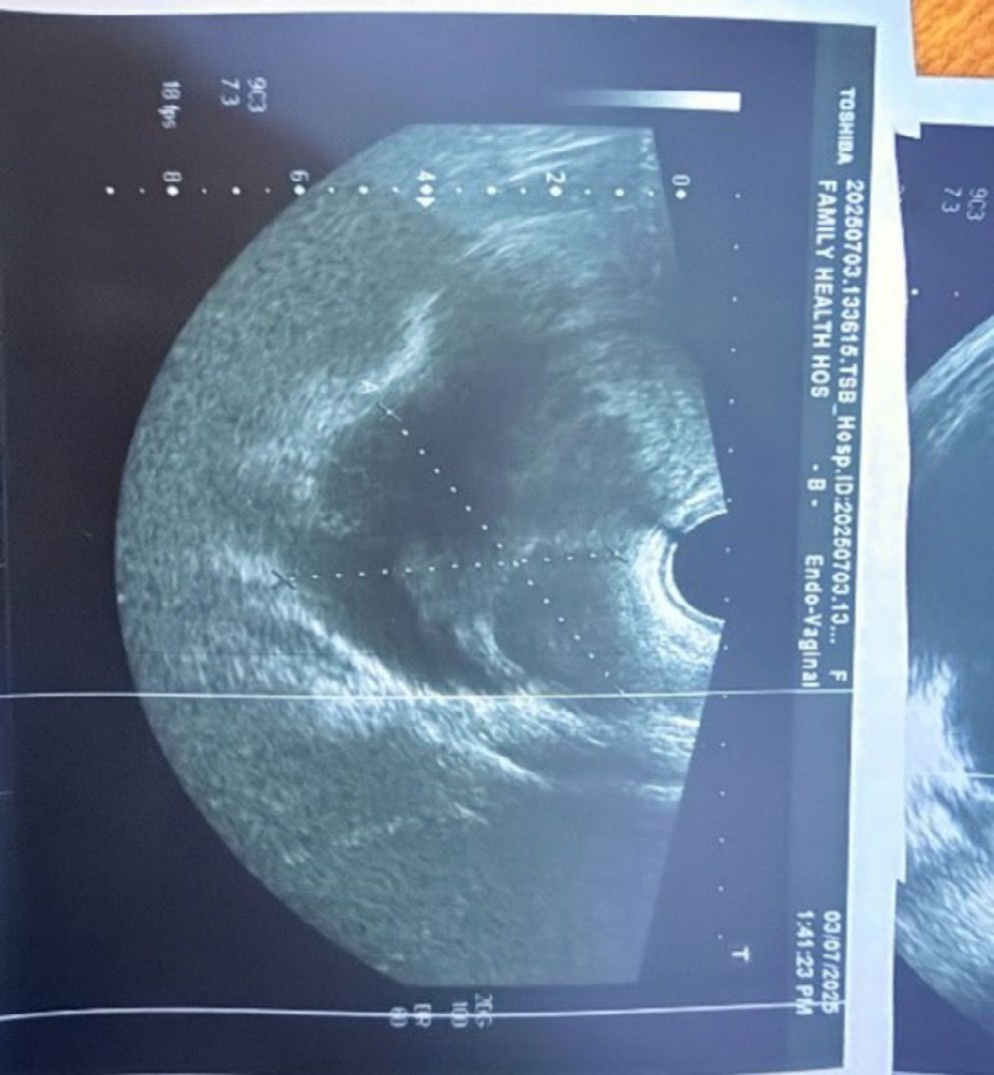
Transvaginal pelvic ultrasound image at 12 weeks' gestation, showing a simple unilocular right ovarian cyst (arrow) adjacent to the intrauterine gestational sac.
